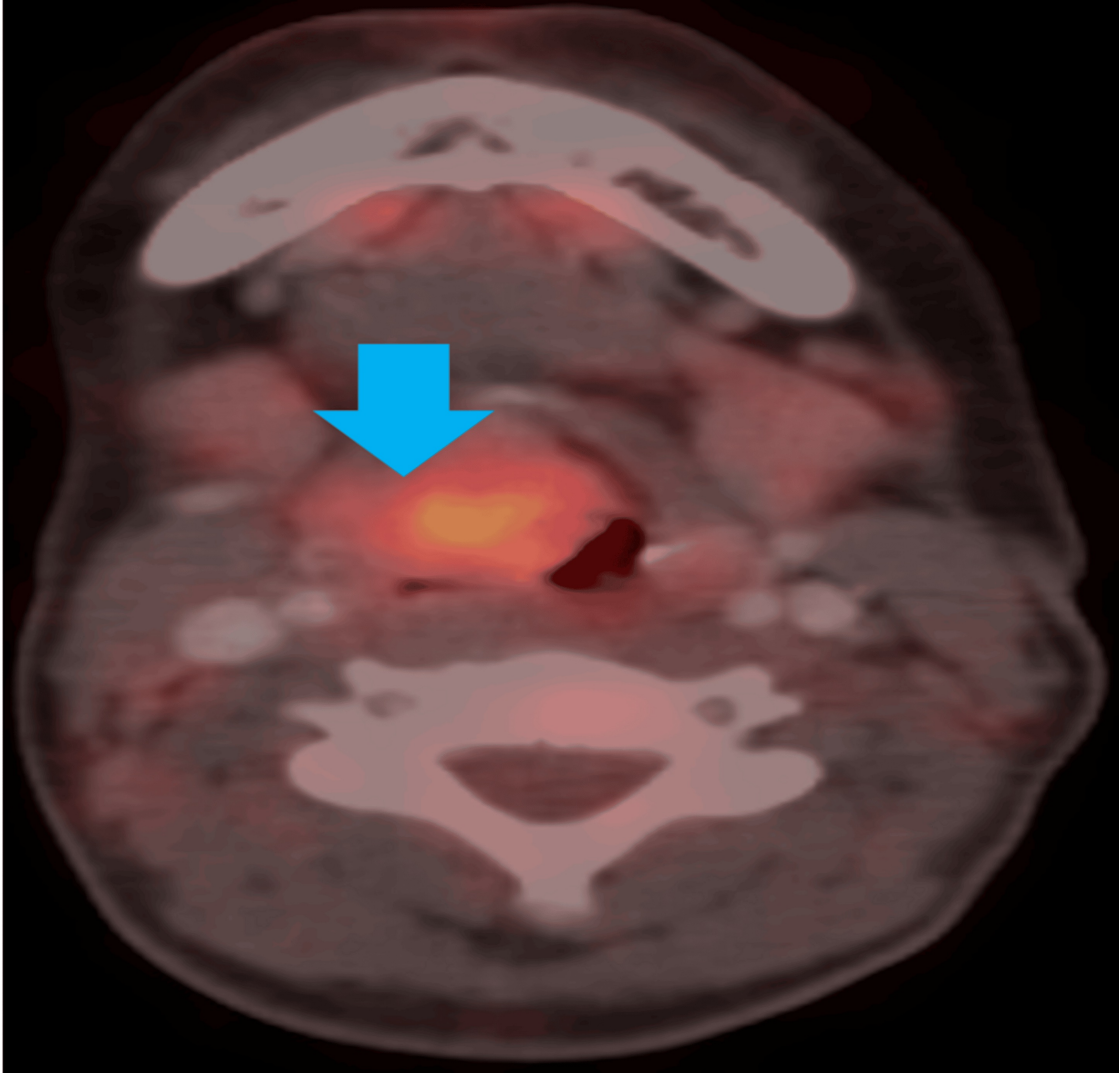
Blue arrow showing large growth in the right side of the larynx involving the vocal cord in the FDG PET/CT scan, size around 2.9 × 2.5 × 2.4 cm, SUVmax: 4.06. SUVmax: maximum standard uptake value; FDG PET/CT: fluorodeoxyglucose positron emission tomography/computed tomography
